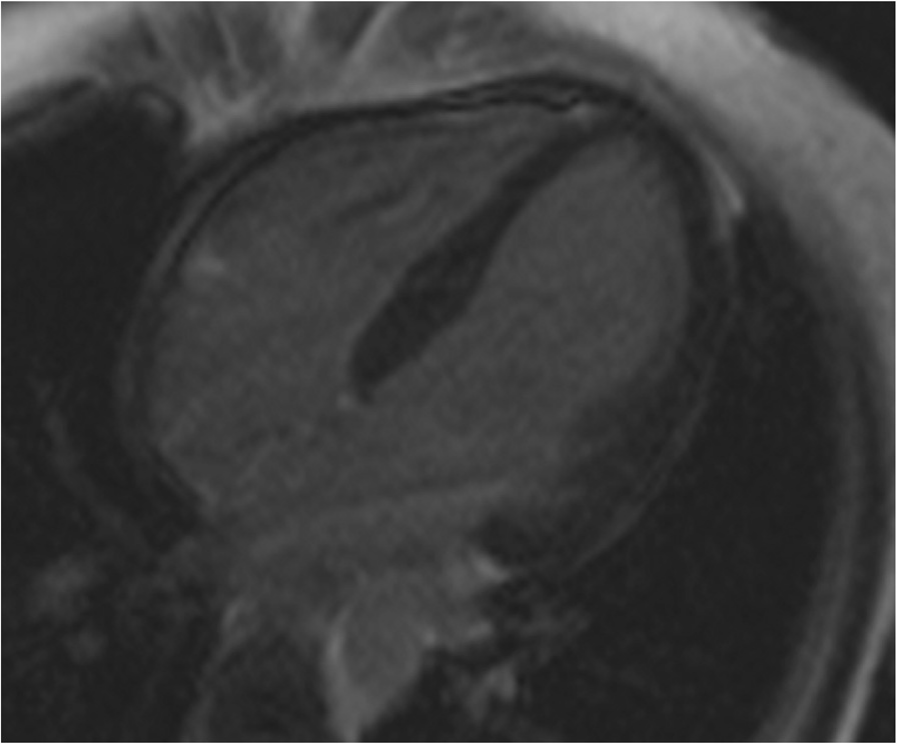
Cardiac magnetic resonance imaging with late gadolinium enhancement in four chamber view, without evidence of tissue abnormalities (scars, patchy fibrosis)
